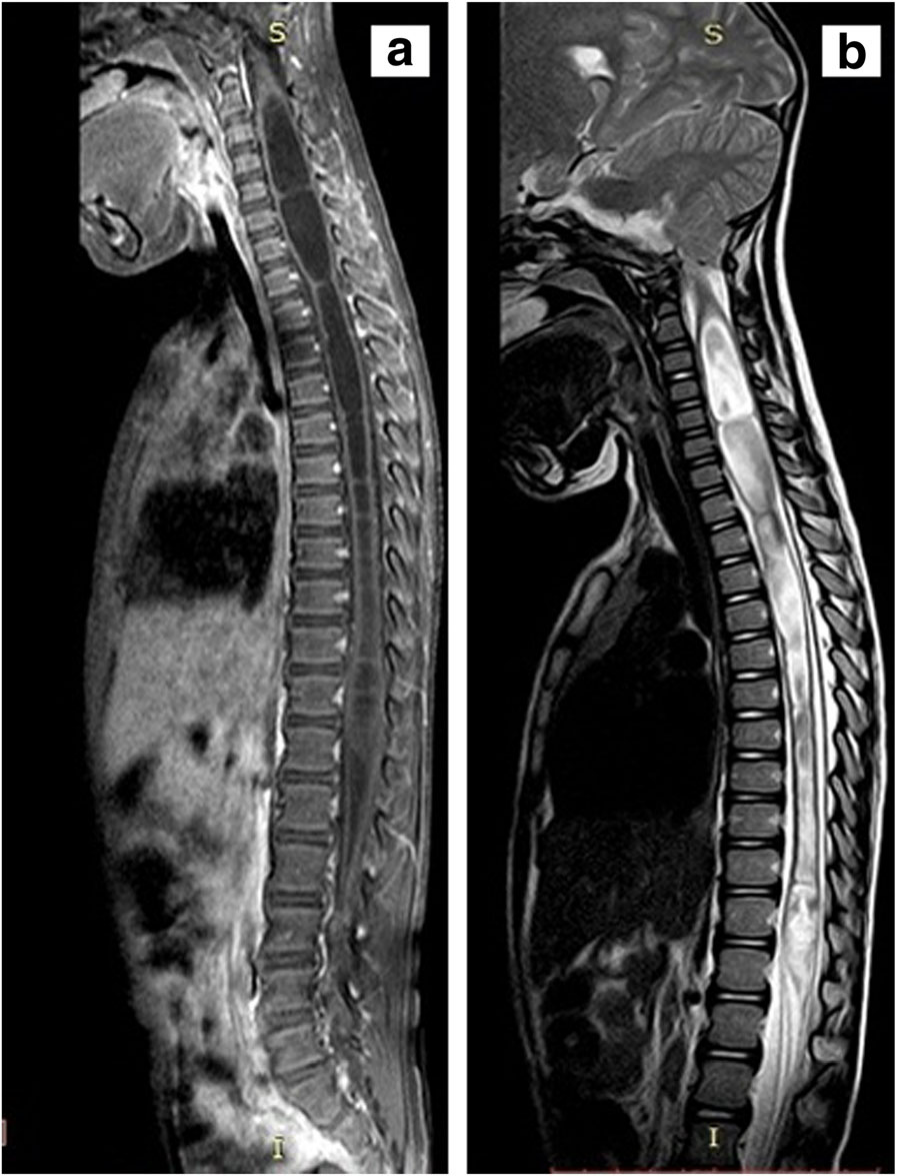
a Sagittal T1-weighted sequence of MRI of thoracolumbar spine showing hypointense central cavitary lesion involving whole cord up to conus medullaris. b Sagittal T2-weighted sequence of MRI of thoracolumbar spine showed central cavitary lesion extending down to the conus medullaris.
